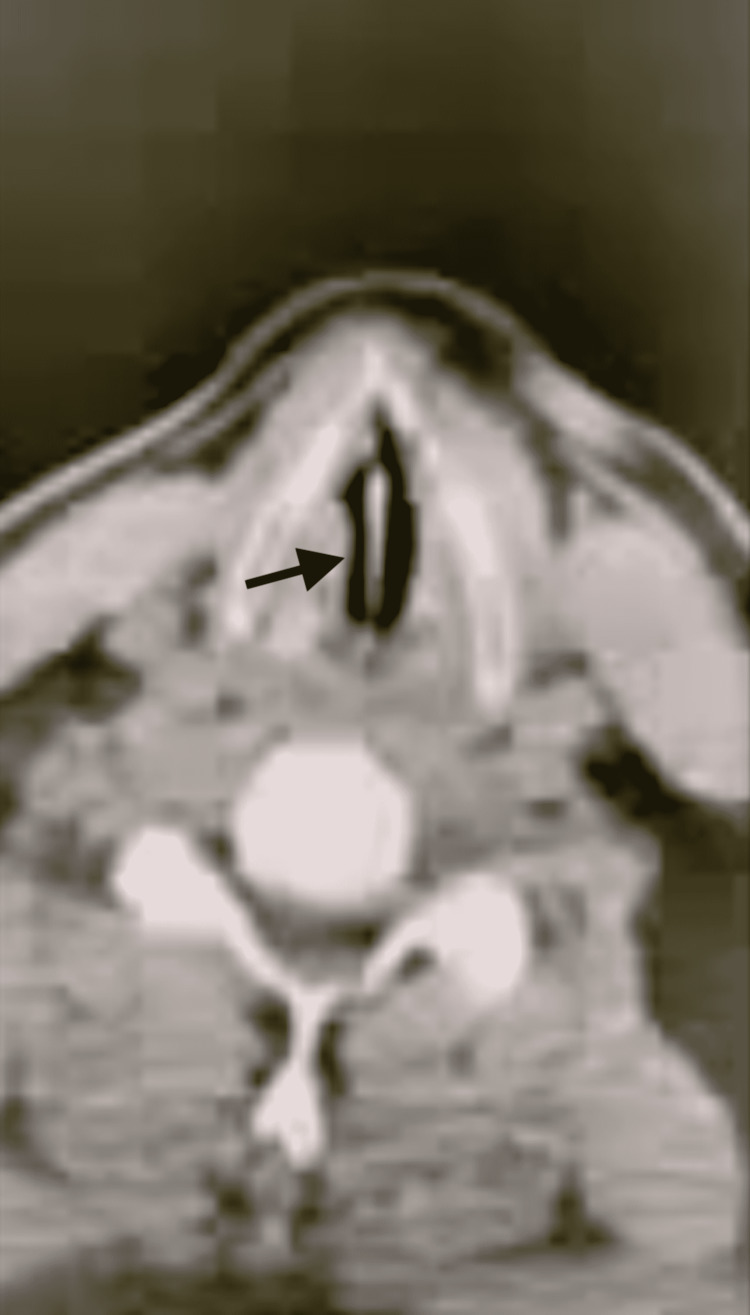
CT of the foreign body in the airway
